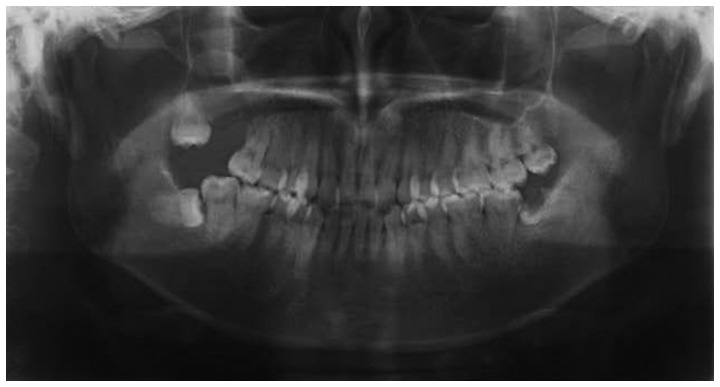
Panoramic radiographic finding. Panoramic radiograph revealing the cystic radiolucent lesion in the right maxilla elevating the floor of the right maxillary sinus, indicating the presence of a cystic lesion or odontogenic tumor of the right maxilla.
